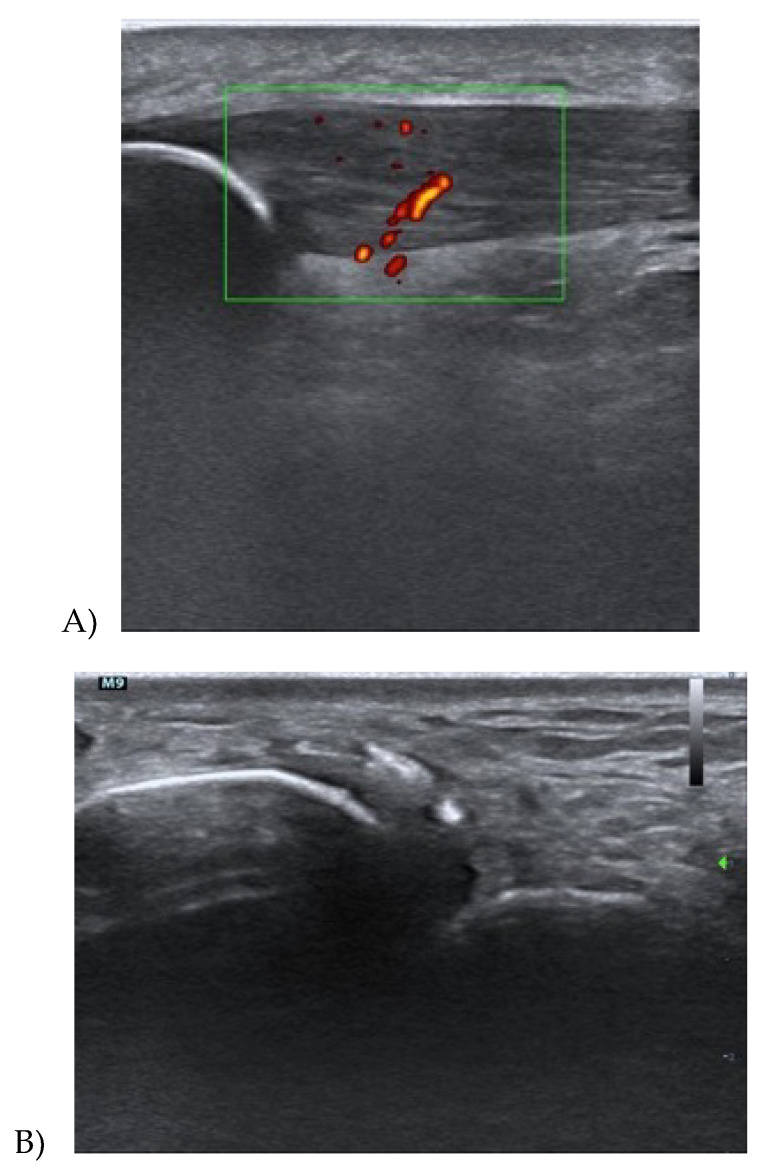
(A,B) Longitudinal section of the patellar tendon and the Achilles tendon showing the considers the primary 3 tendinopathy features: thickness, hypoechogenicity of tendon tissue, and neovascularization. The green box corresponds to the area of the Power Doppler function. The green arrow corresponds to the focus area.
